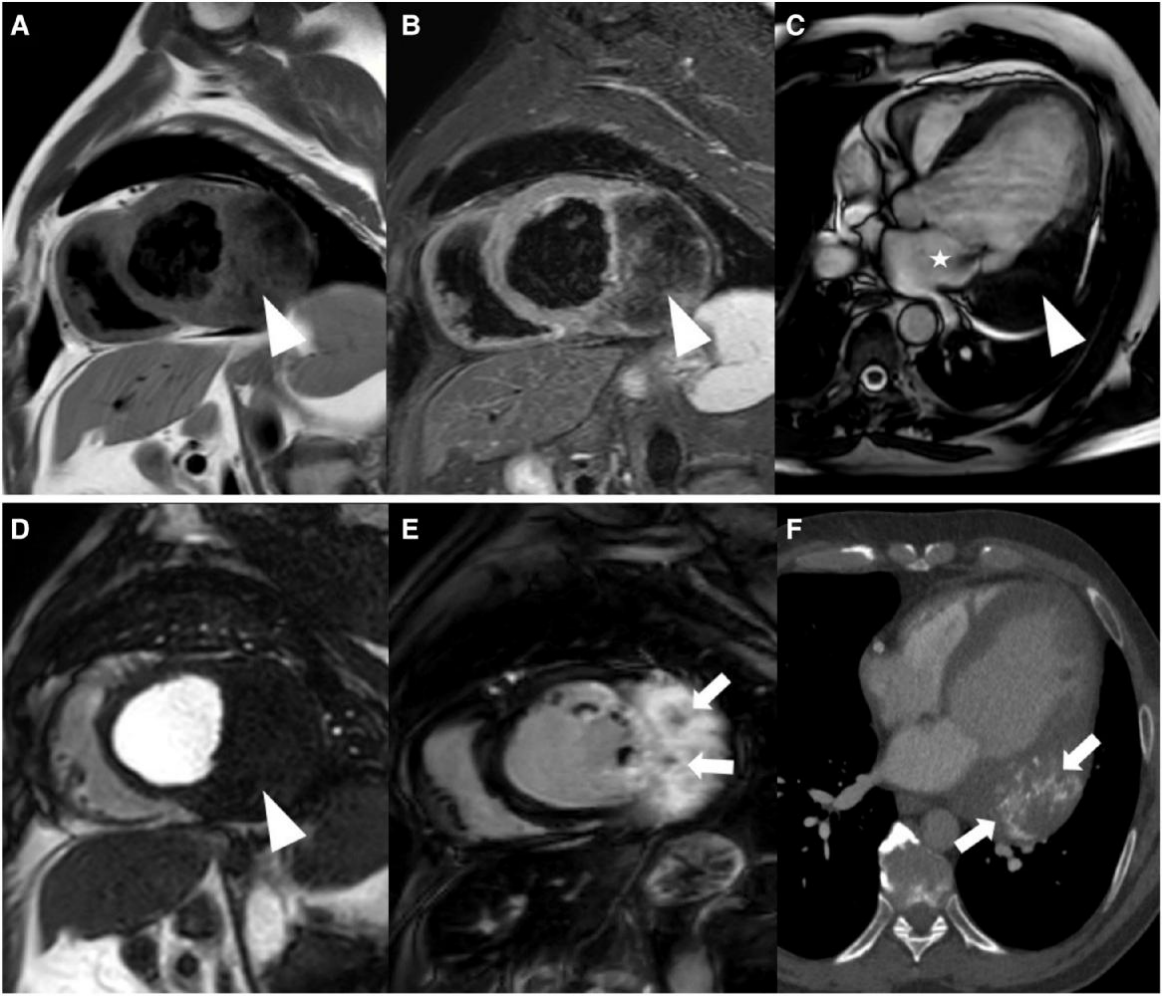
Cardiovascular magnetic resonance showing a semicircular cardiac mass of the left ventricular lateral wall with an iso- to hypointense signal in T1- (*A*, arrowhead) and hypointense signal in T2-weighted (*B*, arrowhead) sequences in short axis. Cine sequence in four-chamber view revealed an iso- to hypointense signal of the mass (*C*, arrowhead) and an attachment to the posterior mitral valve leaflet with associated insufficiency (*C*, asterisk). There was no hypervascularization of the mass in the first-pass-perfusion study in short axis (*D*, arrowhead). Late gadolinium enhancement (LGE) in short axis revealed a strong enhancement of the mass with spotty areas of calcification (*E*, arrows). CT revealed corresponding dystrophic calcifications (*F*, arrows). Overall, these features are consistent with a cardiac fibroma.
